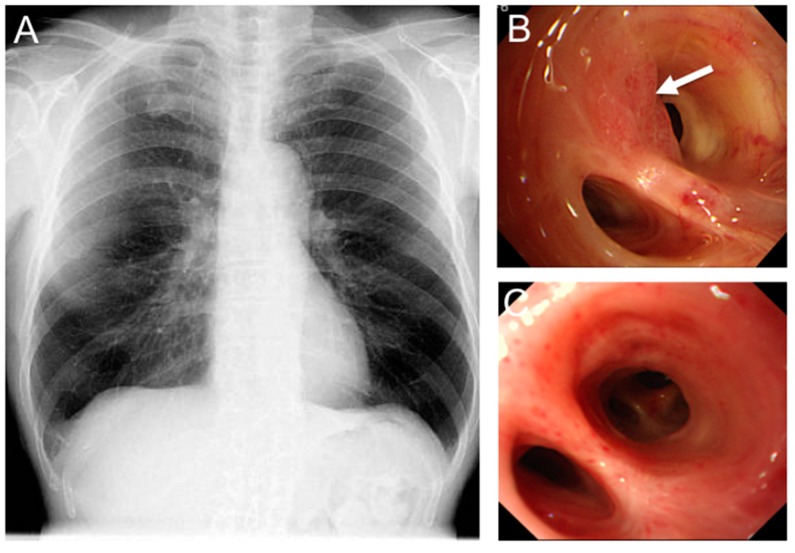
Photodynamic therapy (PDT) for radiographically occult early stage lung cancer. (A) A 65-year-old male presented with abnormal sputum cytology and normal chest X-ray; (B) Bronchoscopy revealed an endobronchial abnormality at the orifice of the left upper division anterior segmental bronchus (arrow). Biopsy was positive for squamous cell carcinoma; and (C) Bronchoscopy six months post PDT (120 J/cm^2^) shows complete response with no residual tumour.
